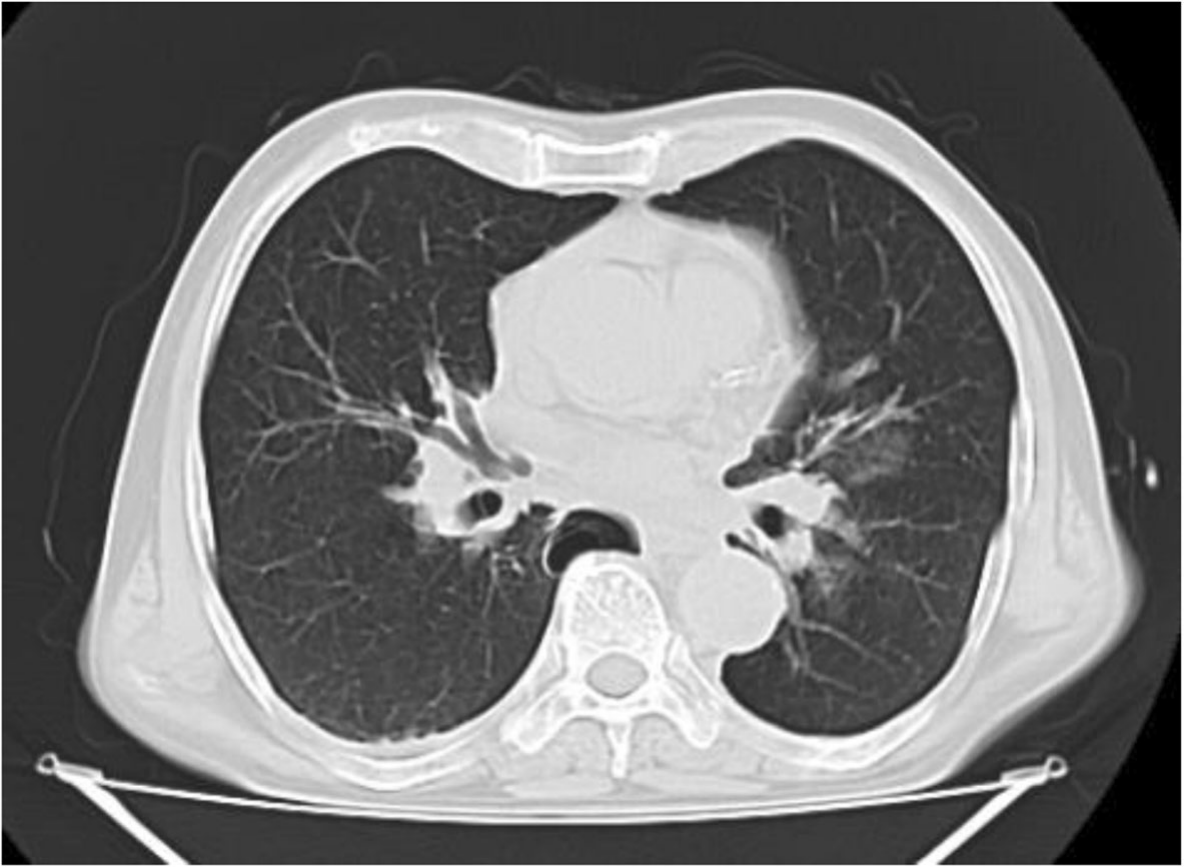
Primary lung CT scan. No pathological lesion was observed on upon arrival lung CT scan of the patient
